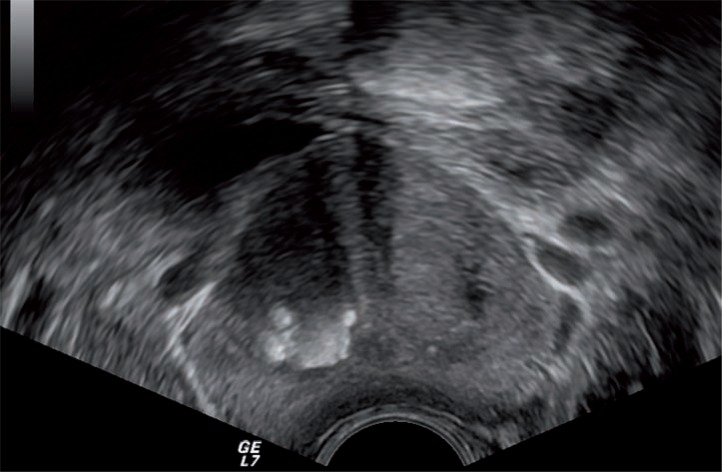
Transrectal ultrasound in axial section of prostate shows marked calcifications with multiple hyperechoic foci and with significant area (≥3mm) with coarse shadow.
